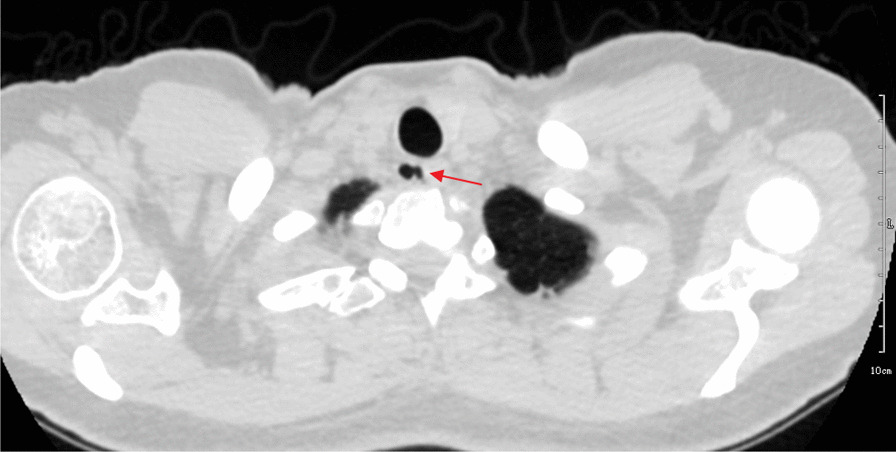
Axial CT image showing a tracheal diverticulum just behind the upper trachea (arrow)
